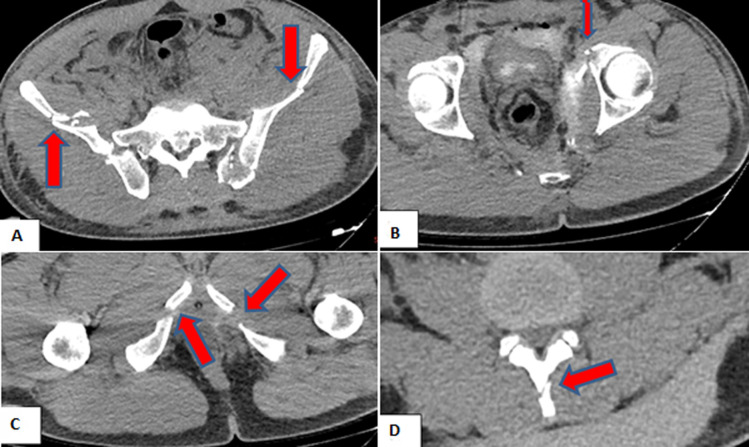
axial plain images of computed tomography showing: A) multiple iliac wings fractures; B) left acetabular fracture; C) fractures of bilateral pubic rami; D) fracture in the lumbar vertebral spinous process (L3) (red arrows)
